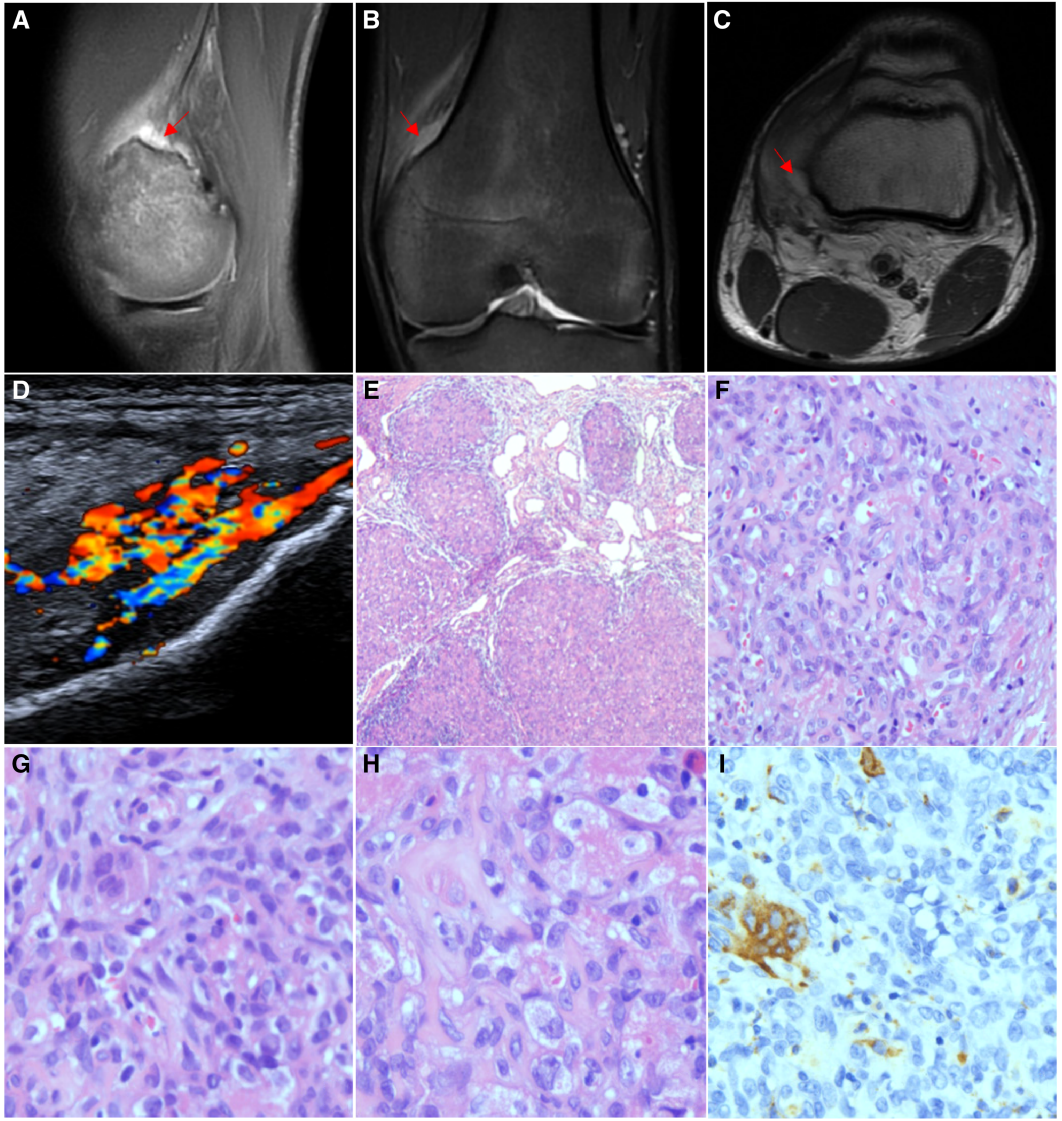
Imaging and pathological features of Case 4. (A) MRI showed an abnormal signal shadow between the posterior upper margin of the left medial femoral epicondyle and the vastus medialis. T2WI showed high signal intensity and a slightly unclear boundary, and the enhanced scan showed obvious enhancement. (B) Lower part of the vastus medialis was blurred, with patchy T2WI − FS hypersignal oedema, which was indistinctly demarcated from the lesion. (C) T1WI + C series shows that the lesion is adjacent to the posterior border of the left medial femoral condyle with local erosion changes by external pressure and a slightly irregular bone cortex in the anterior part. (D) Doppler ultrasound showed an irregular 41 mm*11 mm*27 mm hypoechoic mass between the quadriceps femoris muscle and the medial femoral condyle of the left knee with a vague boundary. (E) Focal haemangioma signs (HE ×40). (F) Microvascular cavities and single-cell cytoplasmic vacuoles were observed between cells (HE ×200). (G) Multinucleated osteoclast-like giant cells (HE ×400). (H) Foam-like histiocytes (HE ×400). (I) CD68 and CD163 are expressed in multinucleated osteoclast-like giant cells (IHC ×200).
